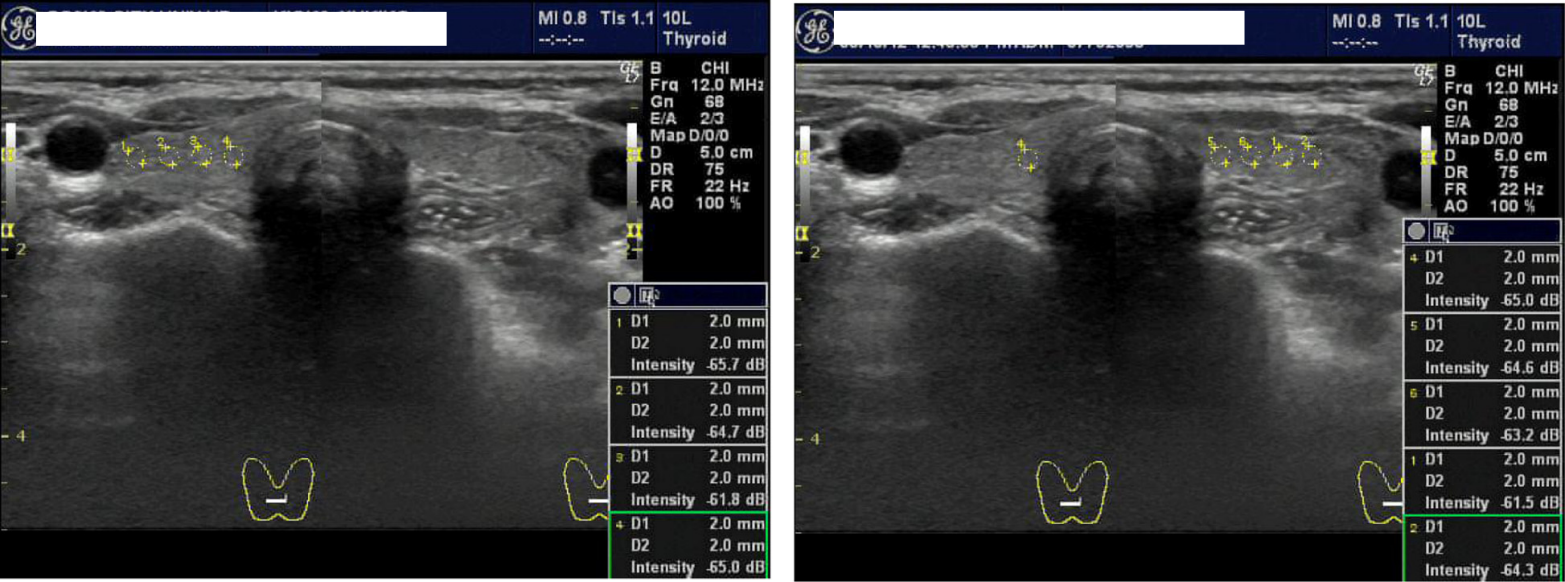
Recording of the ultrasonographic intensities and calculation of the thyroid heterogeneity index (HI). The appropriate sampling points were first determined for measuring. Four open-circle cursors (2 mm in diameter) per half lobe were positioned straight along a horizontal line at the depth of the right common carotid artery adjacent to the thyroid gland. US intensity within each circle was automatically calculated immediately after the cursor was set. HI was defined as the CV (i.e., SD/mean) of eight US intensities.
